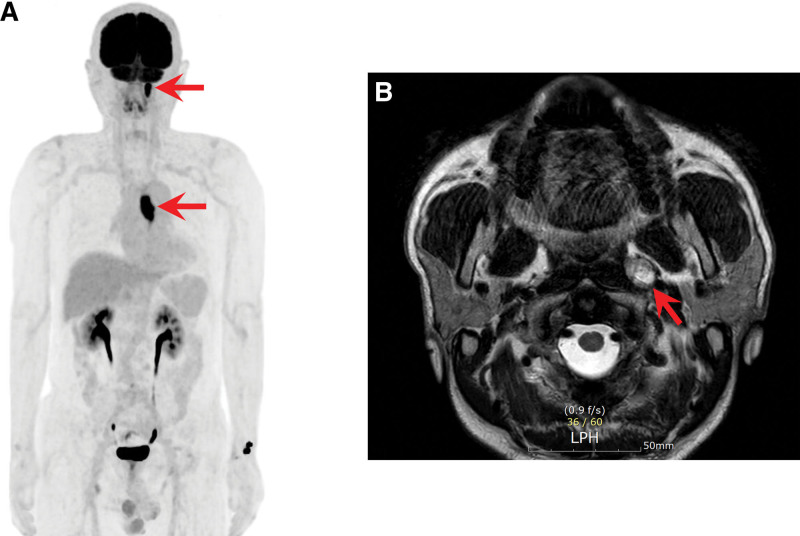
Positron emission tomography and magnetic resonance imaging. (A) Positron emission tomography showed ^18^F-FDG uptake in the mid-thoracic esophagus and left retropharyngeal area. (B) Magnetic resonance imaging of the neck showed a focal, well-defined mass in the left retropharyngeal area. FDG = fluorodeoxyglucose.
